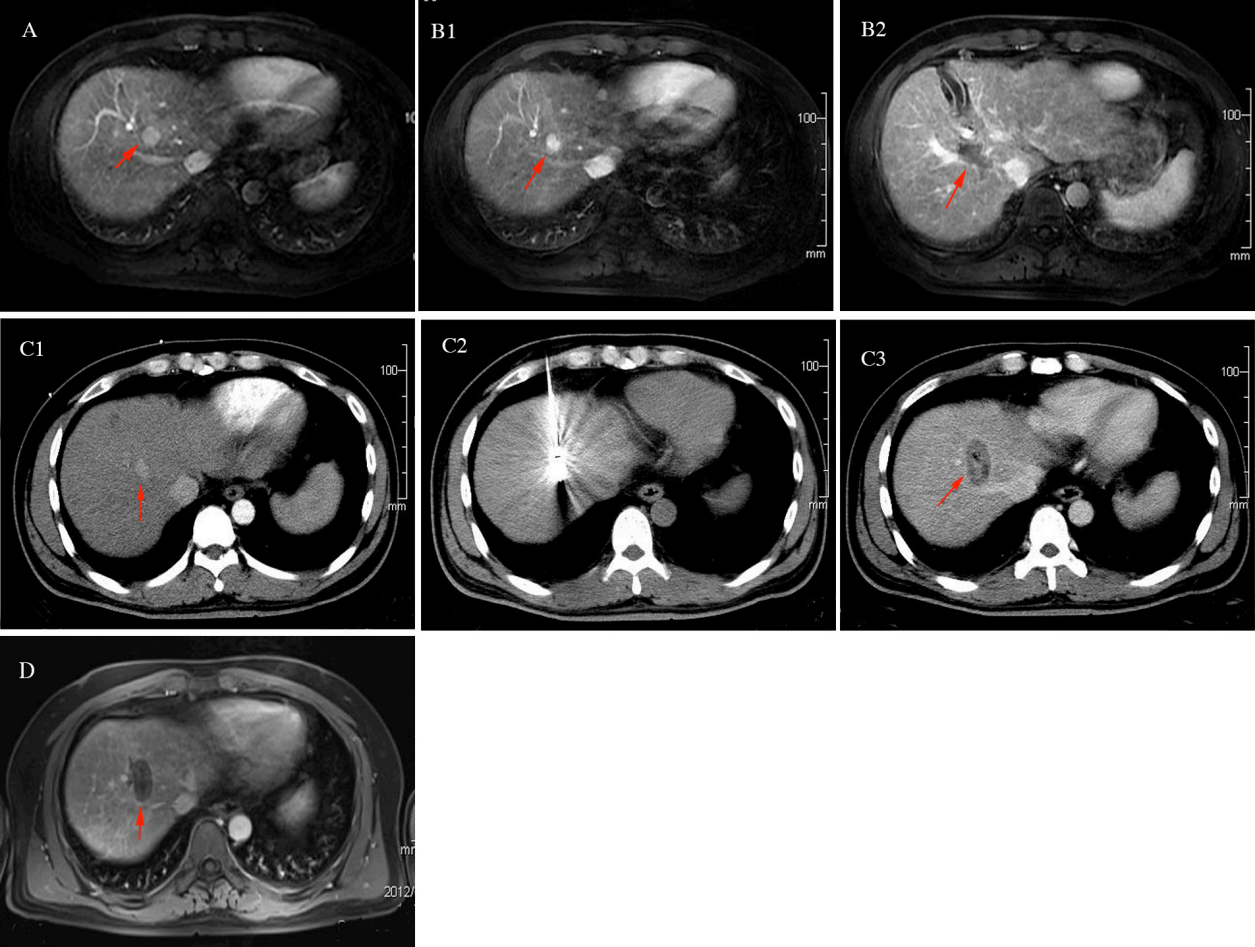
A 68-years-old male patient with primary liver S8 cancer underwent ultrasound-guided microwave ablation previously, but off-target effect appeared one month postoperatively The patients thus underwent CT-guided microwave ablation (MWA) in our hospital. There was no indication of tumor residue or recurrence in the follow-ups. (A) The upper abdomen MRI indicated: liver S8 tumor with the diameter of 1.2 cm (red arrow); (B) Off-target phenomenon occurred after ultrasound-guided MWA: The upper abdomen MRI review: the liver S8 lesion was still there without any significant changes (B1, red arrow), but there was a type of round shaped and low signal area (B2, red arrow) beside the lesion. (C) CT-guided MWA for liver tumor near the diaphragm: C1: Contrast-enhanced CT clearly manifested the liver S8 lesion (red arrow) before MWA. C2: MWA antenna precisely inserted into the tumor during the ablation process, with its needle tip slightly exceeded the edge of the tumor. C3: Contrast-enhanced CT immediately after MWA: ablation area completely covered the tumor (red arrow), without tumor residue. (D) The upper abdomen MRI review one month postoperatively: tumor achieved complete response without residue.
